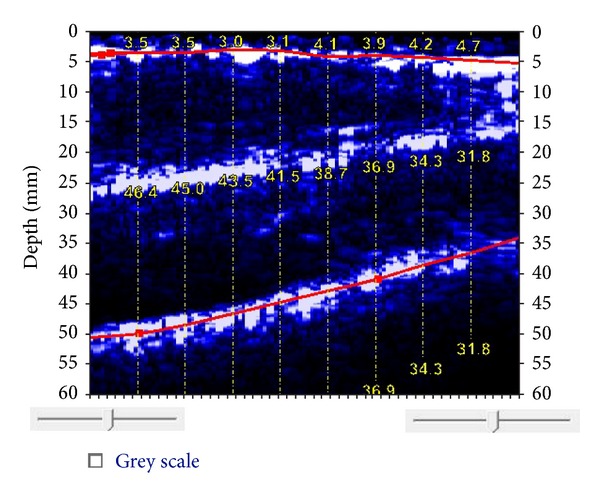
BodyMetrix ultrasound image of thigh. The top line indicates the subcutaneous fat-muscle interface (average thickness of 3.69 mm). The bottom line indicates the muscle-bone boundary. The muscle thickness ranges from 32.0 mm to 46.6 mm. The white layer in the center is the boundary of the rectus femoris and vastus intermedius.
